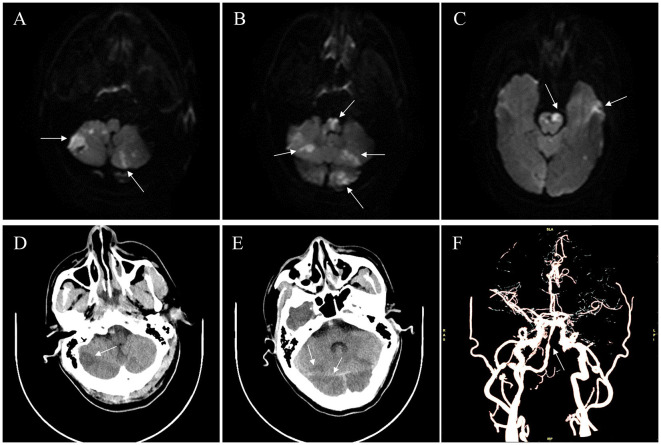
Neuroimaging follow-up. (A–E) Postoperative follow-up revealed multiple small newly developed infarcts (arrows) in the cerebellum and medulla oblongata on diffusion weighted imaging and computed tomography (CT). (F) Postoperative CT angiography confirmed the general patency of the BA trunk, with slight stenosis (arrow).
